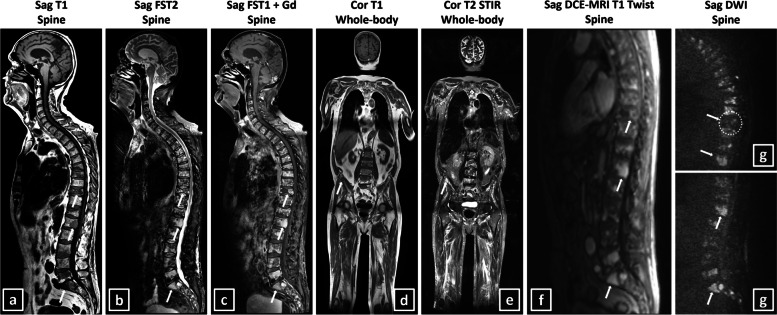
Overview of the 1.5-Tesla MRI scanning protocol using whole-body conventional anatomical MRI sequences (a–e), spinal dynamic contrast-enhanced MRI (f), and spinal diffusion-weighted imaging (g). A 77-year-old male patient with double hit high-risk IgGκ multiple myeloma with Salmon-Durie Plus and Revised International Staging System (second revision, R2-ISS) stadium II is presented, which was unresponsive to therapy and passed away 1.8 years after diagnosis. Regarding SLIM-CRAB criteria, a monoclonal bone marrow plasmacytosis of 50%, a light-chain involved/uninvolved ratio of 42, a total number of 19 focal MRI lesions > 5 mm (largest: 22 mm), a normocalcaemia, a mildly reduced renal function (glomerular filtration rate 60 mL/min/1.72 m^2^, CKD stage G2) and a macrocytic anemia were observed. Suspected focal lesions of more than 5 mm in the 10th thoracic, 1st lumbar, and 1st sacral vertebra and the right iliac bone (white arrows) and diffuse abnormal signal intensities on all sequences are observed. A combined skeletal score of 11/13 with a combined focal and diffuse bone marrow invasion pattern can be observed. The b1000 diffusion-weighted images show severe diffusion restriction in all vertebrae and in the focal lesions of the 1st lumbar and 1st sacral vertebra (white arrows in g). The suspected focal lesion in the 10th thoracic vertebra does not show diffusion restriction (white dotted circle) or contrast enhancement, depicting its benign character due to a recent compression fracture. The spinal dynamic contrast-enhanced MRI sequence, 50 s after gadolinium contrast administration (Gadovist 7.5 mL, gadobutrol 1.0 mmol/mL, 0.1 mmol/kg, Bayer), shows intense and fast contrast uptake in the entire spine and especially in the focal lesions (white arrows in f). *Cor* coronal, *DCE-MRI* dynamic contrast-enhanced magnetic resonance imaging, *DWI* diffusion-weighted imaging, *FS* fat-saturated, *Gd* gadolinium, *sag* sagittal, *STIR* short tau inversion recovery, *T1* T1-weighted, *T2* T2-weighted
